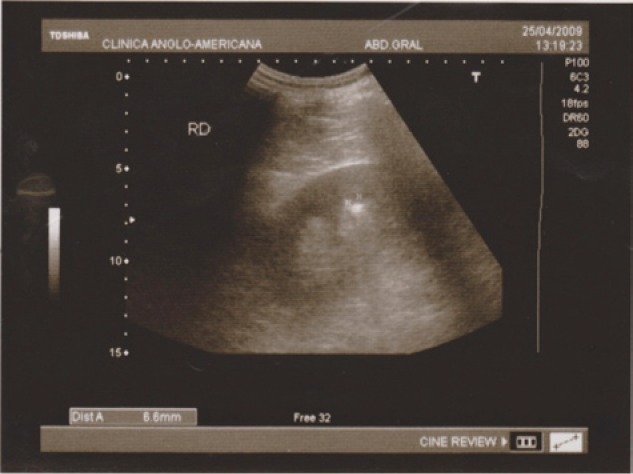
Renal sonography showing uncomplicated nephrolithiasis.
